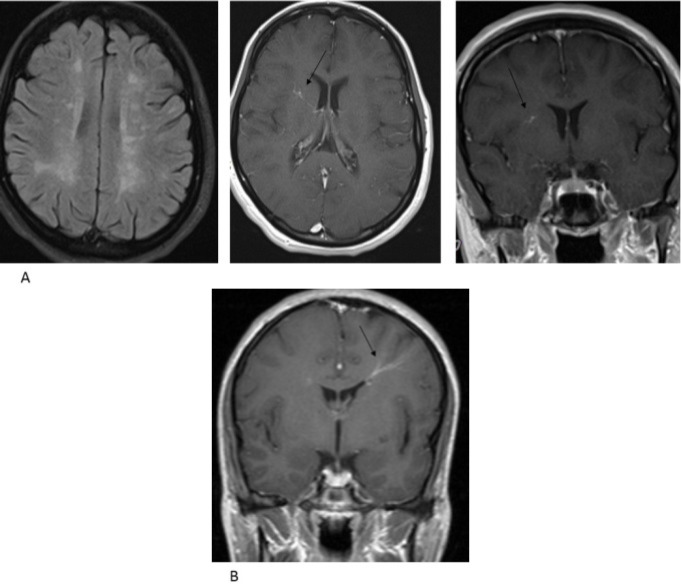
Developmental venous anomaly (DVA) in a patient with multiple sclerosis (MS). Axial fluid-attenuated inversion recovery image shows MS (multiple sclerosis) plaques and post-contrast T1-weighted images show enhancing vessels joining the collecting vein (arrows) (A), Developmental venous anomaly (DVA) in a control subject. Post-contrast T1-weighted image shows enhancing vessels joining the collecting vein (arrow) (B).
